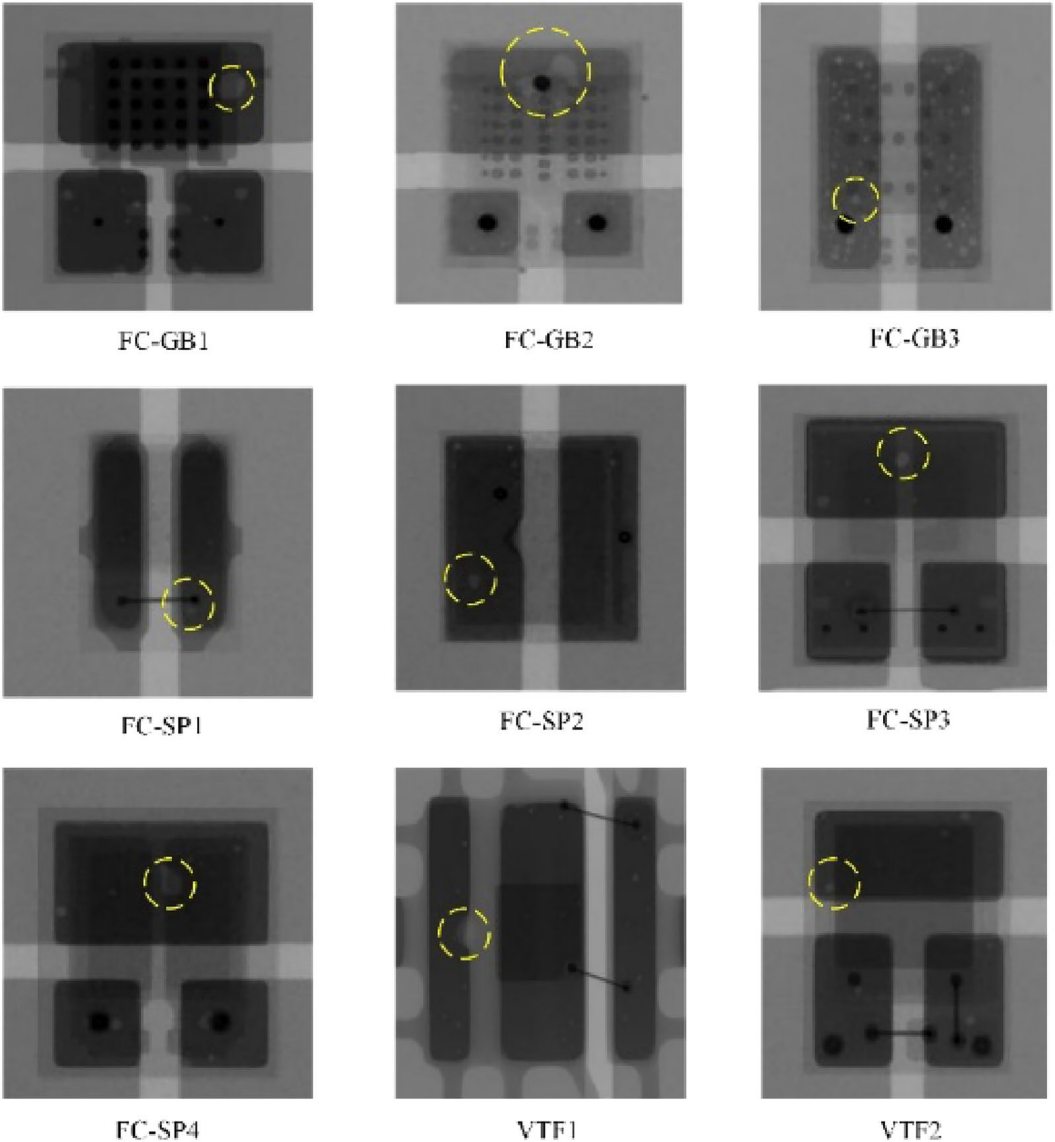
X-ray images of 9 kinds of LED chips and some hollow defects are shown.
